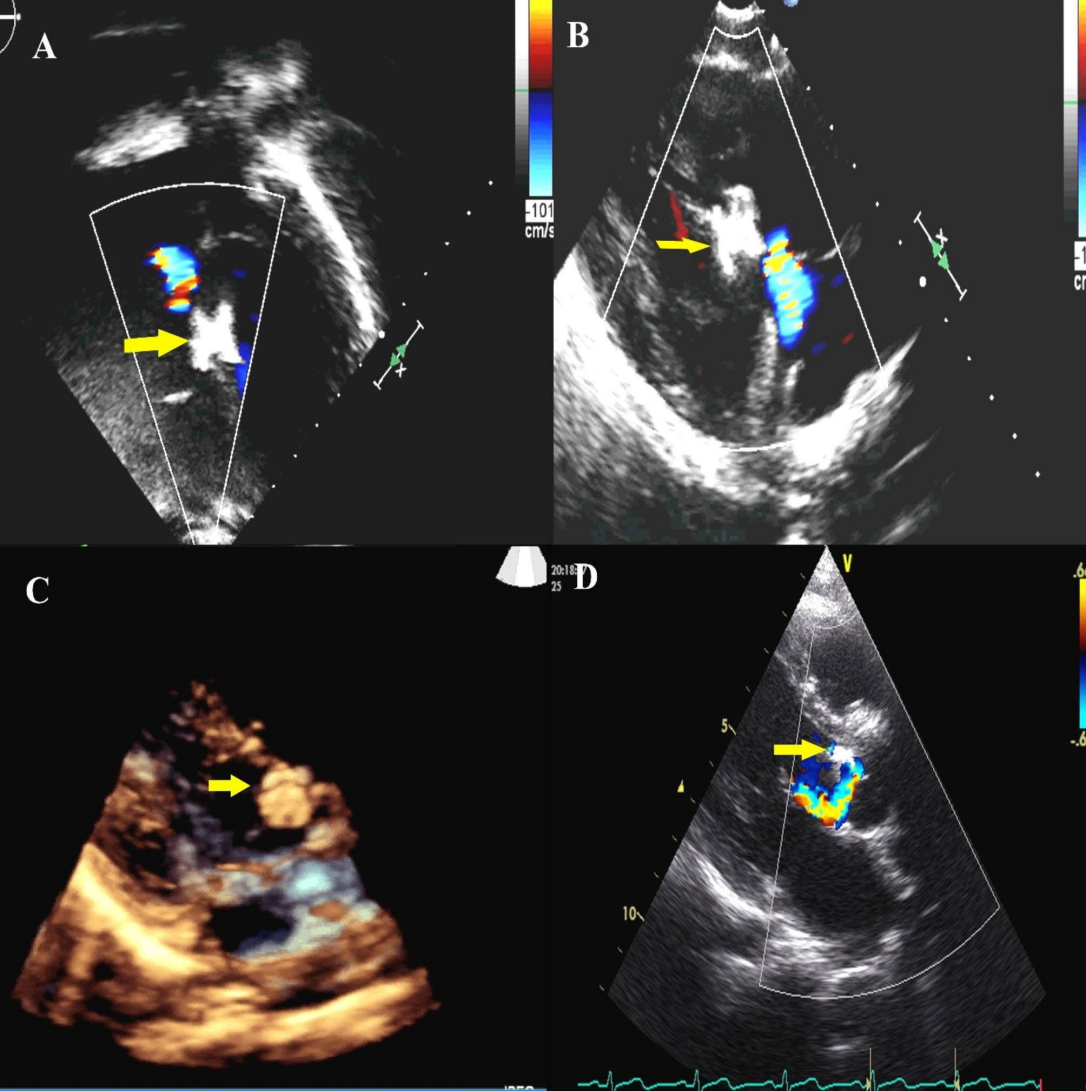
Long-term follow-up using transthoracic echocardiography: (A, B) Two-dimensional four-chamber view and left parasternal long-axis view (in rightward and inferior angulation toward right hip) demonstrating mild tricuspid regurgitation caused by Hyperion muscular device (yellow Arrow) used to close a high muscular outlet VSD (C), Three-dimensional parasternal long-axis view of ADO I is seen slightly protruding in the LVOT causing minimal aortic regurgitation and (D) Long parasternal view demonstrating aortic regurgitation caused by a device in closing the perimembranous VSD.
